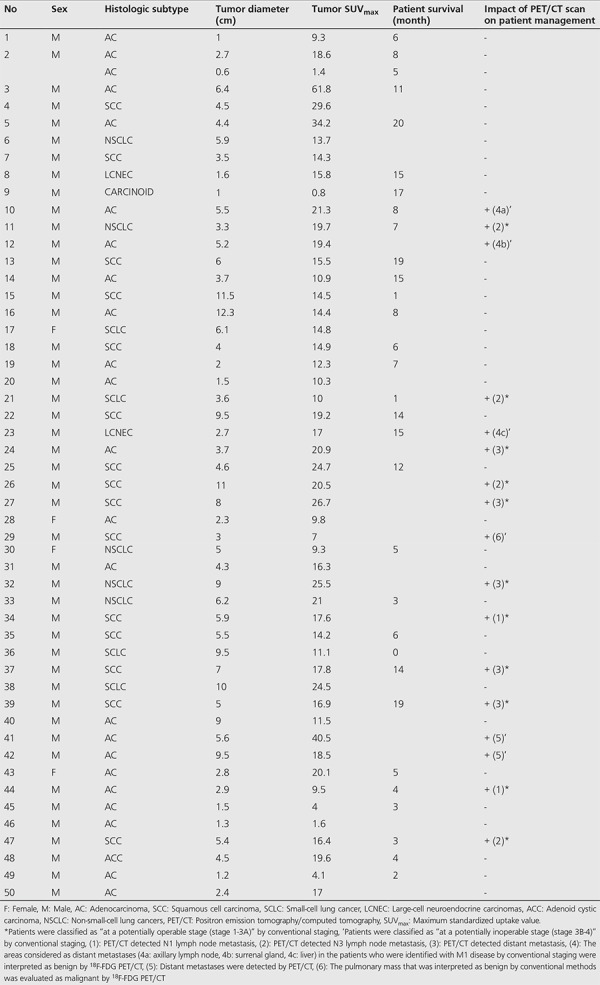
Patient-tumor characteristics and the impact of positron emission tomography/computed tomography on patient management
